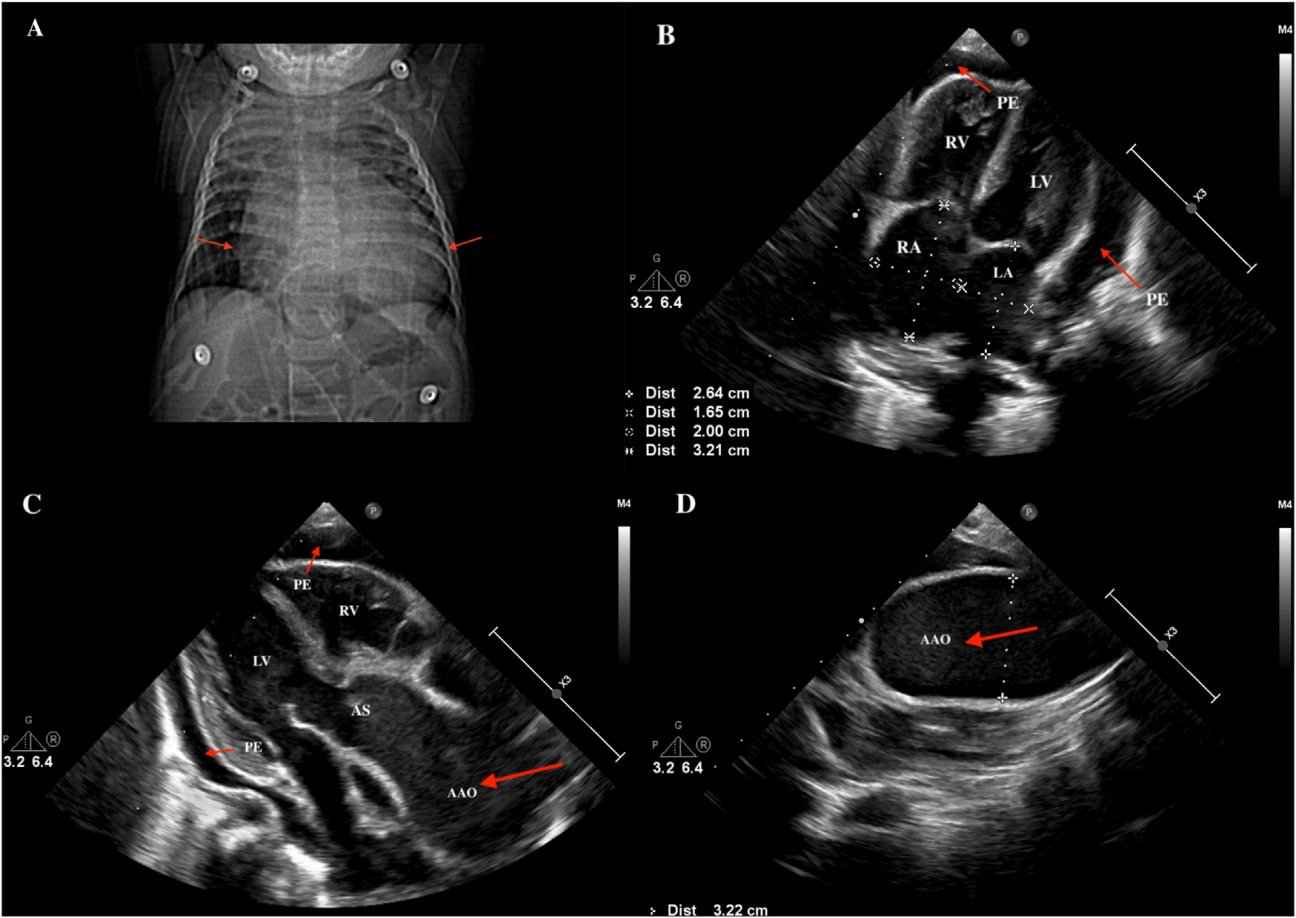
The Results of chest X-ray and cardiac color ultrasound. (A) Chest X-ray showed an enlarged cardiac boundary (red arrow). (B) cardiac color ultrasound indicated enlargement of the left and right atria (RA 20*32mm, LA 17 mm) and pericardial effusion (red arrow). (C) Ascending aortic aneurysmal dilation and pericardial effusion (red arrow). (D) Aneurysmal dilated ascending aorta (red arrow). PE, pericardial effusion; RV, right ventricle; LV, left ventricle; RA, right atrium; LA, left atrium; AS, aortic sinus; AAO, ascending aorta.
